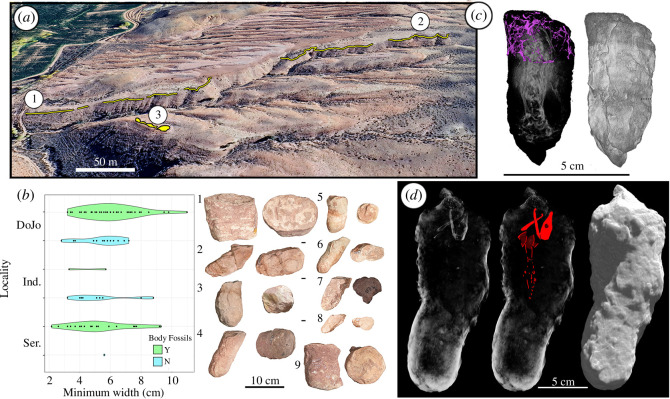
Burrow localities, size distribution, and morphotypes of the Serendipity beds (yellow lines), upper Jelm Formation, near Dubois, WY, and collection localities: (*a*) (1) Serendipity, (2) DoJo, and (3) Independence. (*b*) Plot of representative burrows (*n* = 88) from the three localities (data in the table 1) demonstrating size distribution and general morphology of burrows (1, UWGM 7191; 2, 7188; 3, 7193; 4, 7194; 5, 7196; 6, 7189; 7, 7192; 8, 7195; 9, 7190). (*c*) Neutron tomography rendering of UWGM 2204 (morphosource.org; ID:000609370) containing a skeleton and rhizoliths within burrow, purple = rhizolith network from top 2 cm of burrow (left), volume rendering (right). (*d*) Micro-computed tomography rendering of UWGM 7438 with a partially articulated skeleton (left), highlighted skeleton (middle), burrow cast volume rendering (right).
